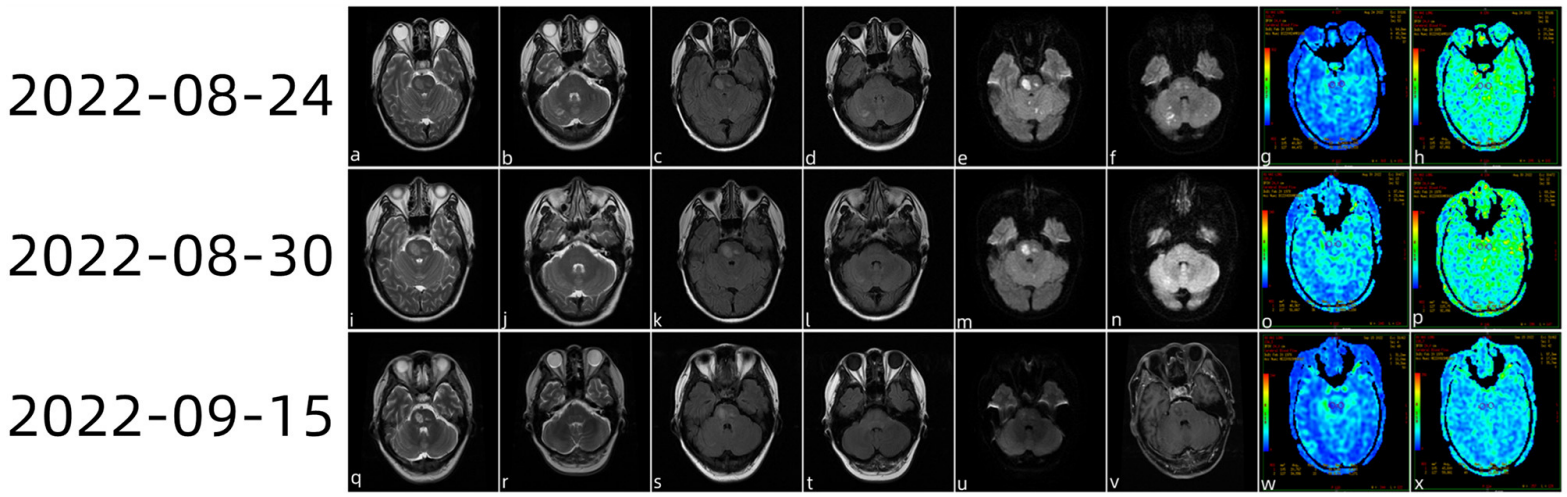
Head magnetic resonance imaging (MRI) plain scan 3.0 examination diagnosis (a–f) on 24 August 2022 showing multiple acute foci in the brainstem and bilateral cerebellar hemispheres. ASL (g, h) showed a mild reduction in the perfusion of the lesions. On 30 August 2022, the cranial MRI (i–n) showed that the bilateral cerebellar acute infarction had improved and that the brainstem acute infarction had reduced slightly. ASL revealed slight hypoperfusion when PLD (o) was 1.5 s and the cerebral stem perfusion was similar to that of the contralateral side at 2.5 s (p). On 15 September 2022, the patient came to the hospital for review (q–x); brain stem lesions were smaller than those of half a month ago, while magnetic resonance enhancement of the brain showed (v) heterogeneous enhanced foci in the brain stem.
